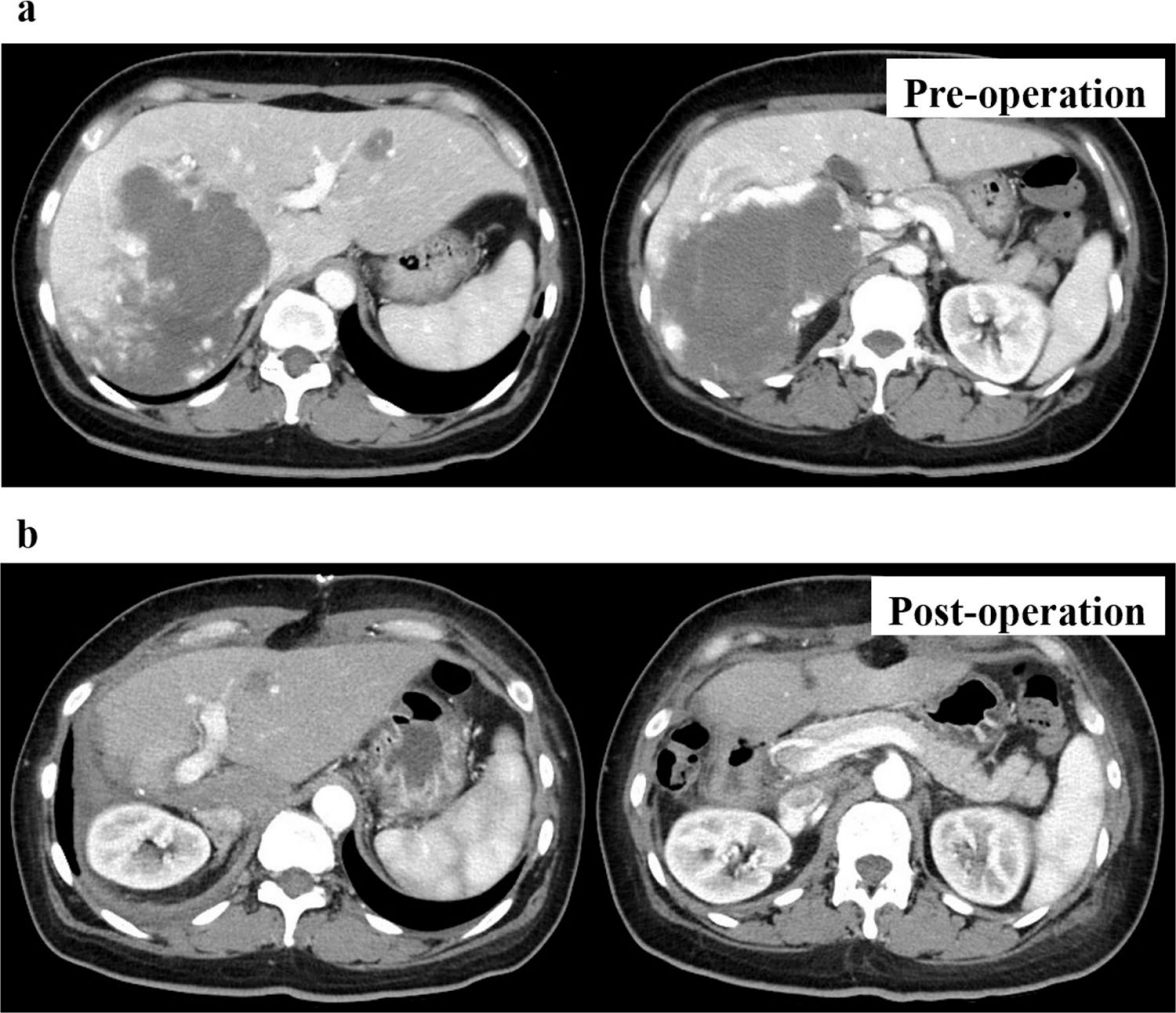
Abdominal contrast-enhanced CT (arterial phase). a Pre-operation. b Post-operation. The giant hepatic hemangioma was successfully removed, and no complications were associated with the surgery. *CT* computed tomography
